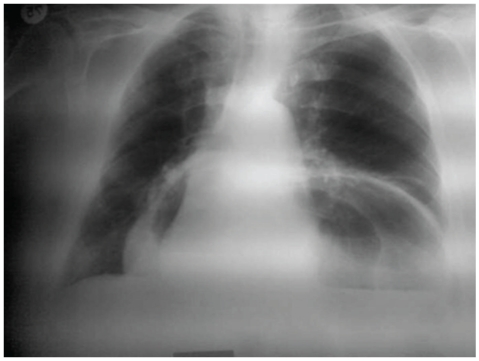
Preoperative chest roentgenogram showing a large hiatal hernia.
